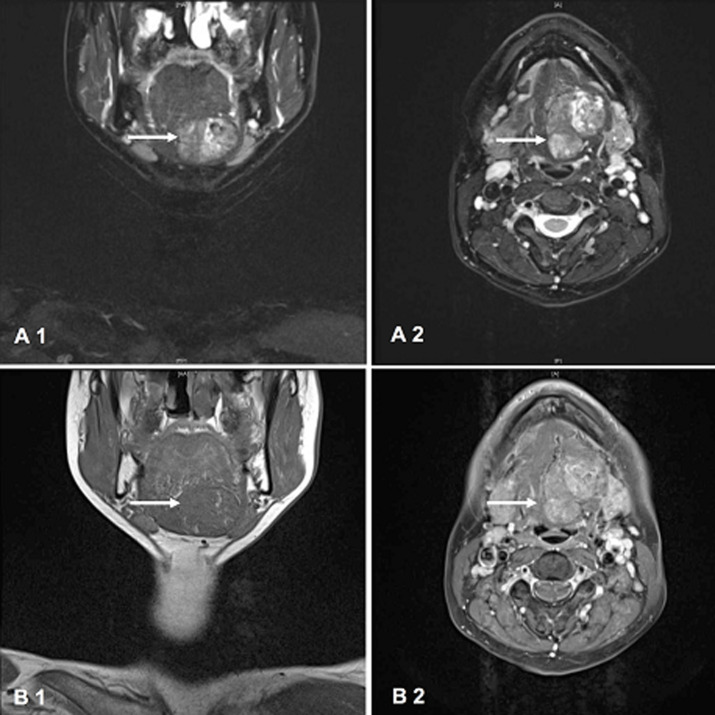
MRI scan showing a submental mass (white arrow): A1) T2-weighted (coronary); A2) T2-weighted (axial); B1) T1-weighted (coronary); B2) T1-weighted (axial)
